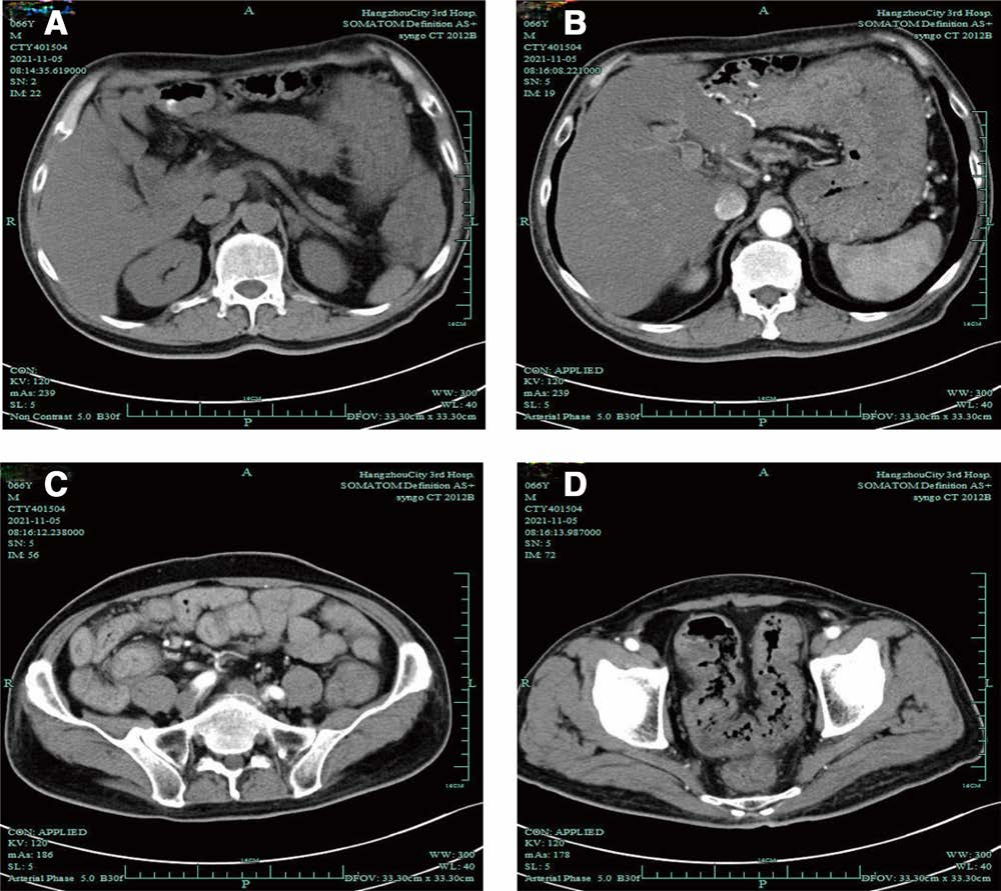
(A–D) Enhanced CT of the whole abdomen suggested more colonic contents, extensive thickening of the intestinal wall, obvious enhancement of the mucosa after enhancement, part of the intestinal lumen was narrowed, and the peripheral fat interstitial exudation was not obvious, and no enlarged lymph node shadow was seen. After gallbladder surgery; after pancreatic head and neck resection. CT = computed tomography.
